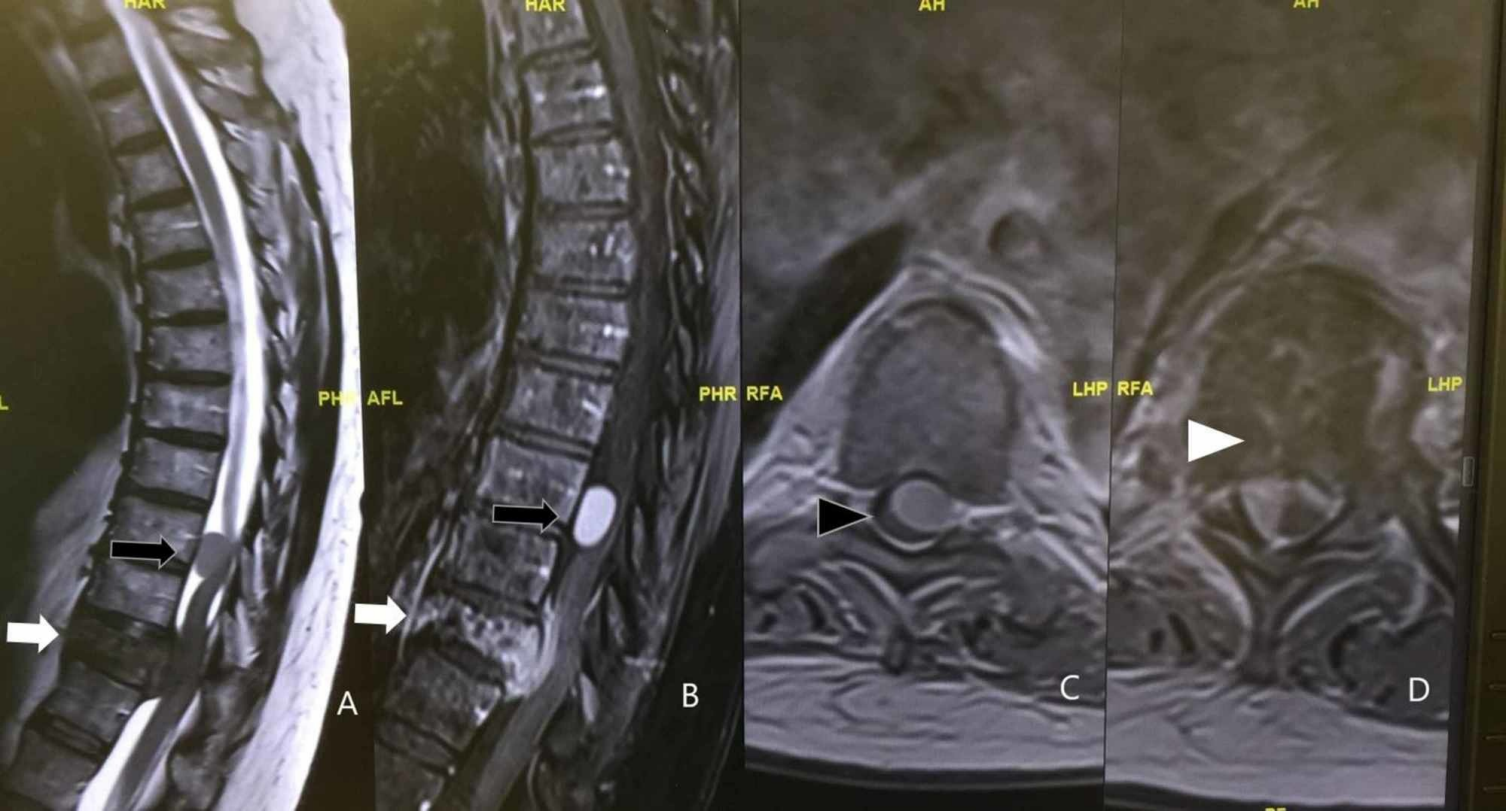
Sagittal T2-weighted without contrast (A) and sagittal T1-weighted MRI scan with gadopentetate dimeglumine contrast (B) demonstrate an intradural, extramedullary T1 enhancing lesion at T9 (black arrow) and a simultaneous destructive process of the T11 vertebral body (white arrow) with T1 enhancement of the adjacent anterior epidural space with severe spinal stenosis. Axial T1-weighted MRI scan with gadopentetate dimeglumine contrast demonstrate a intradural, extramedullary T1 enhancing lesion (black arrowhead) at the level of T9 (C) and a destructive process of the T11 vertebral body (white arrowhead) with enhancing collection on the right anterior epidural space at T11 with severe spinal stenosis (D).
